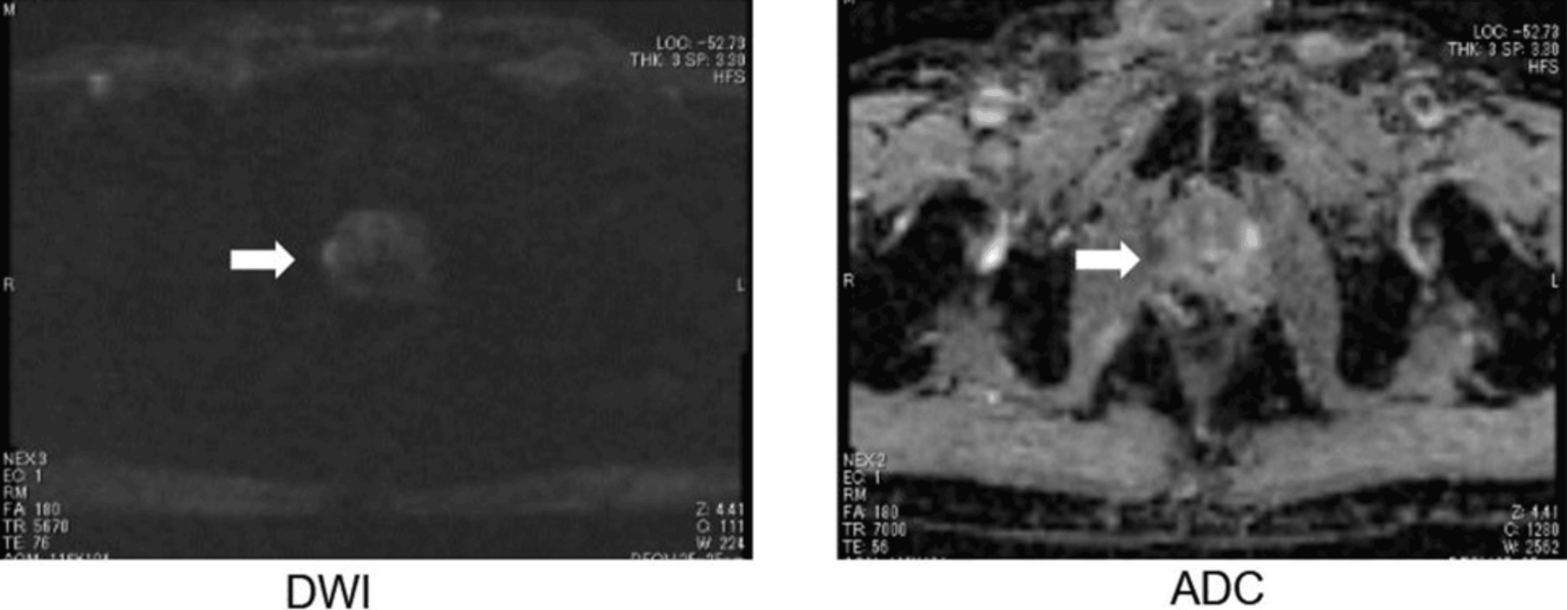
MRI imaging of the prostate at initial diagnosis Left: diffusion-weighted imaging (DWI); Right: apparent diffusion coefficient (ADC) map
